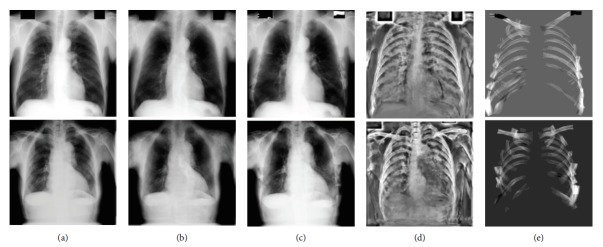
Decomposition results of two chest radiographs from the JSRT data set: (a) the original chest radiographs (“JPCLN063” and “JPCNN071”); (b) the reconstructed soft tissue images by the use of our method; (c) the bone-suppressed images by the use of the gradient modification method; (d) the reconstructed bone images by the use of our method; (e) the estimated bone structures by the use of the gradient modification method.
